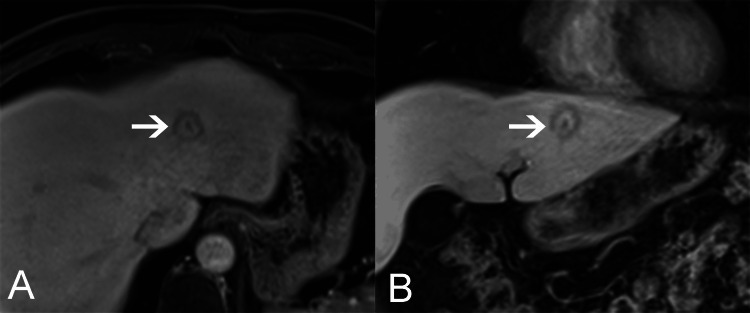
Gd-EOB-DTPA-enhanced MRI findings of BDA in axial (A) and coronal (B) views. The tumor showed a ring of high intensity (arrows) in the hepatobiliary specific period and low signal peripheral and central of the tumor.
